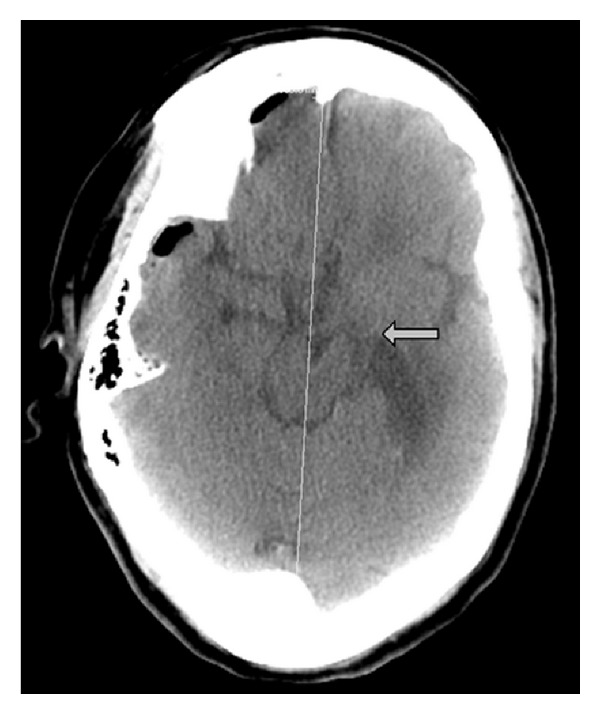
CT of the head after craniotomy and evacuation of subdural hematoma showing persistent midline shift of the midbrain with deformity of crus cerebri (marked by arrow).
